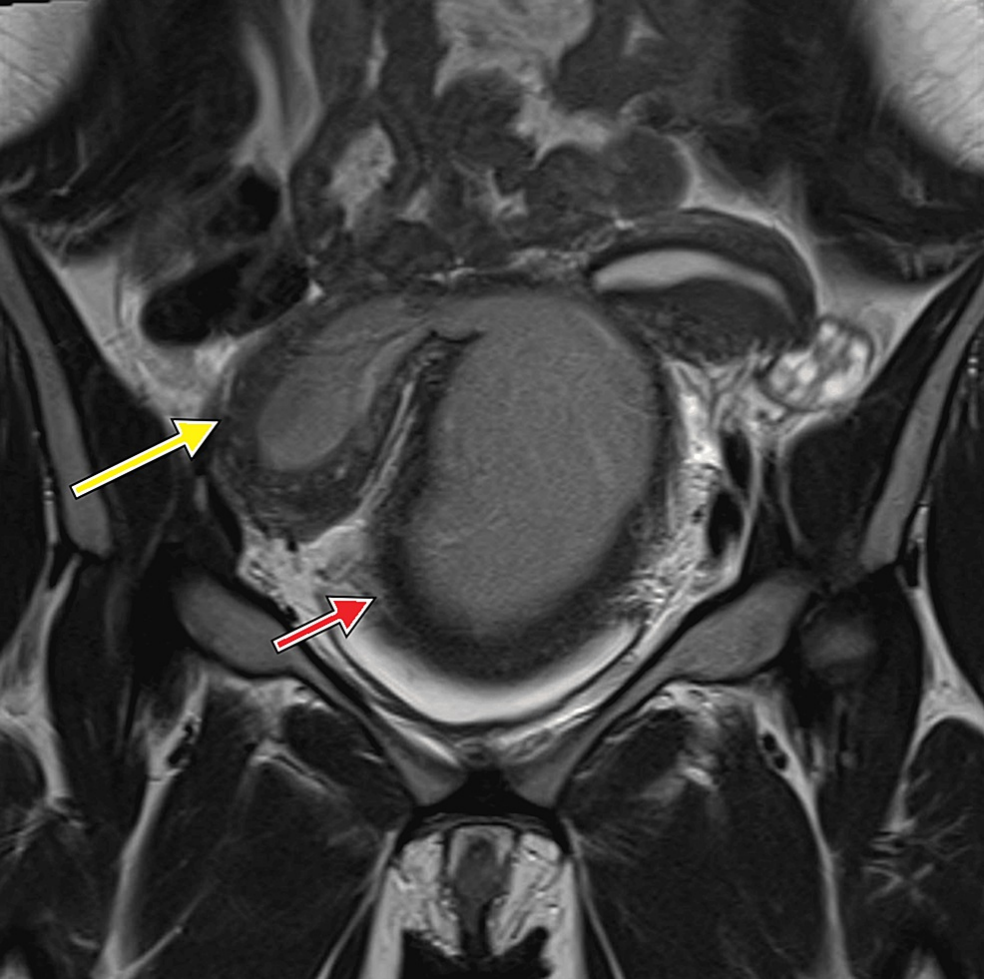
Coronal MR image showing a didelphys uterus with a dilated right endometrial cavity (yellow arrow) and dilated right hemivagina (red arrow). MR: magnetic resonance
